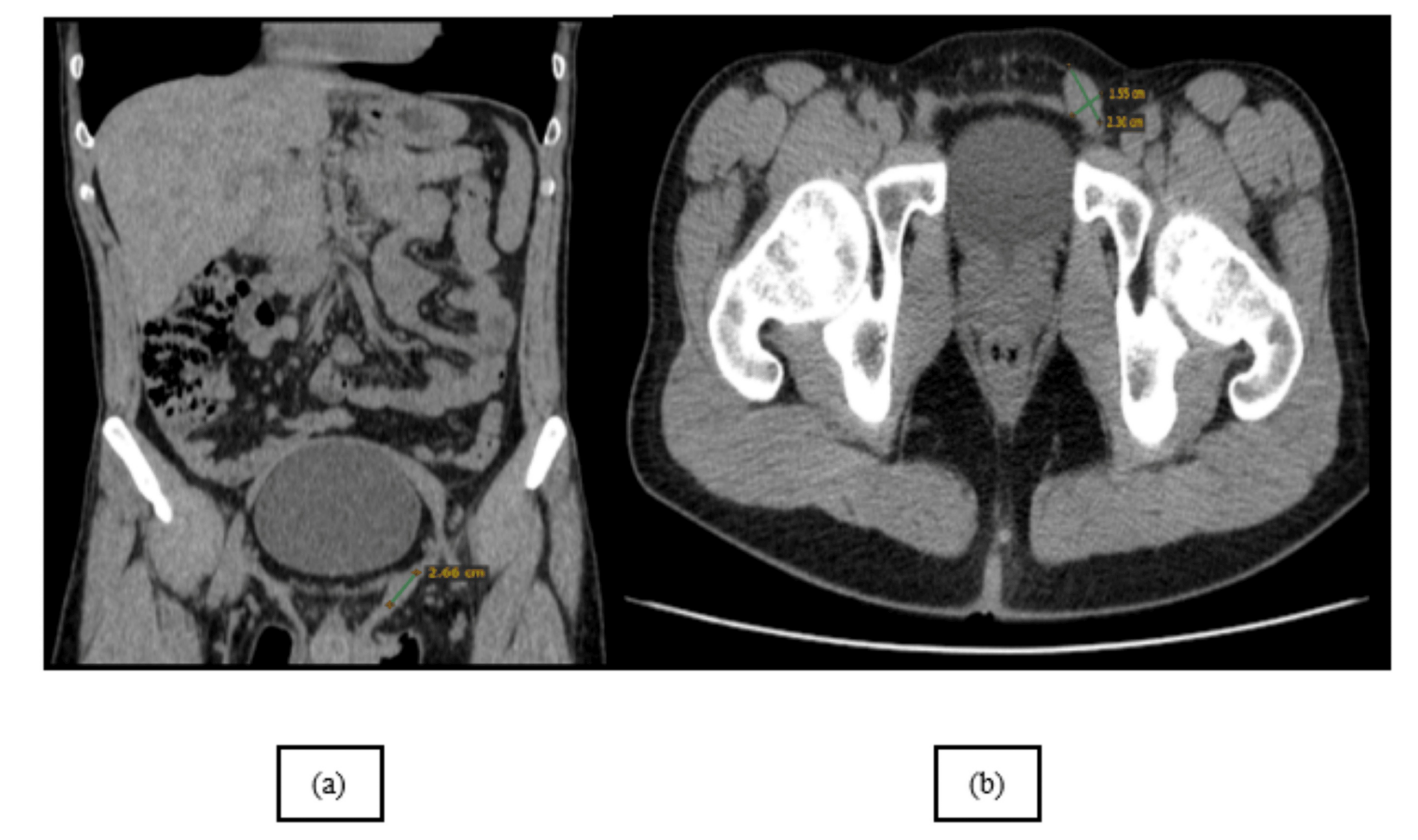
Coronal and axial NECT scans of the pelvis show a small round, isoattenuating mass in the upper part of the left inguinal canal. NECT: non-contrast-enhanced computed tomography This figure is the original work of the authors. Patient consent for the use of the image was obtained, as mentioned in the patient consent form.
